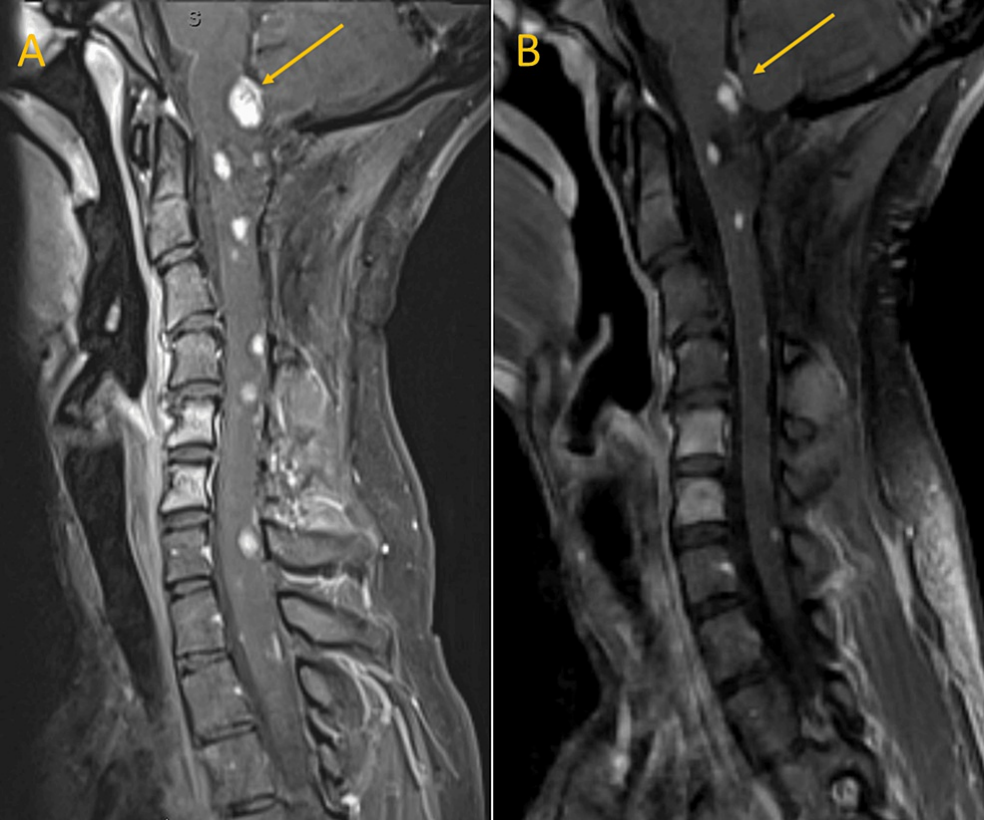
Initial magnetic resonance imaging (MRI) of the cervical spine (T1 post-contrast) (A) Surveillance MRI showing multiple enhancing lesions throughout the neuraxis with the largest lesion at the cervicomedullary junction measuring 9 x 9 x 12 mm (yellow arrow). (B) Three-month follow-up MRI post the belzutifan treatment showing an interval decrease in size to 5 x 7 x 9 mm (yellow arrow).
